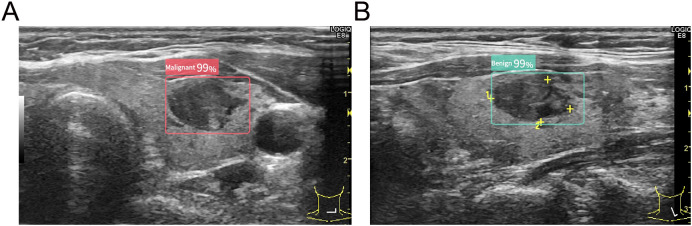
Representative case of equal malignant-risk output in both imaging planes. (A) Transverse-plane and (B) longitudinal-plane ultrasound images of a thyroid nodule in a 52-year-old woman show identical malignant-risk output (99%) on both planes. According to the predefined algorithm, the final AI classification was “Possible Malignant.” Postoperative pathology confirmed medullary thyroid carcinoma.
